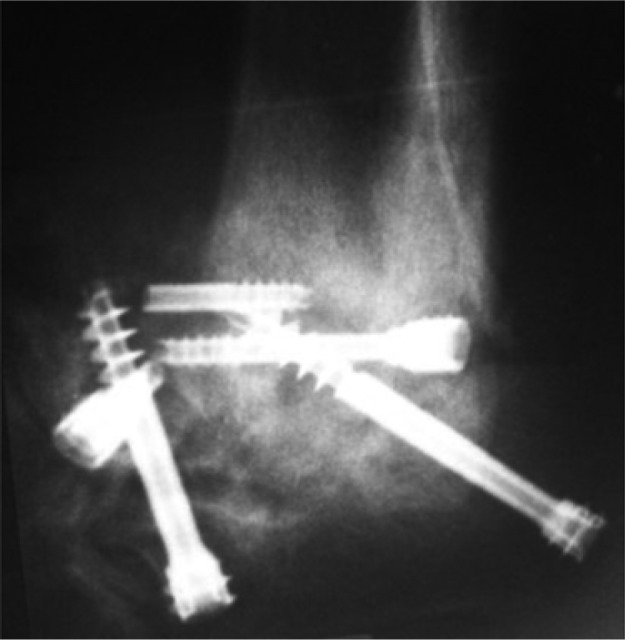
Post-operative plain radiograph showing the mechanical assembly failure and progression.
